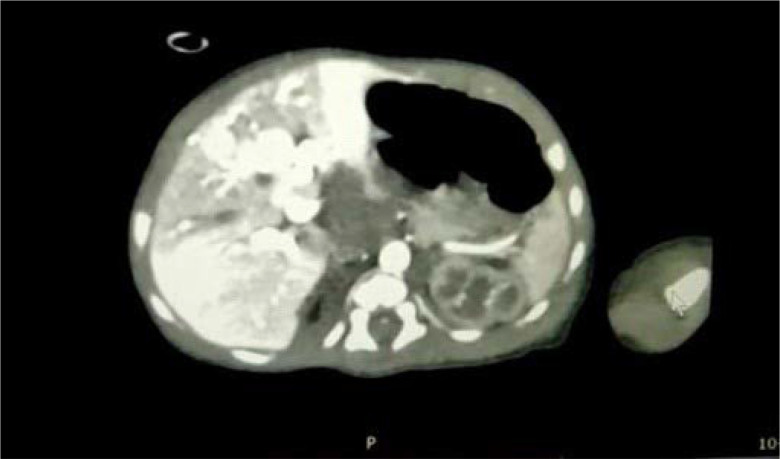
Contrast-enhanced CT scan showing mild enhancement or attenuation similar to that of adjacent parenchyma on delayed images and dilated celiac trunk.
